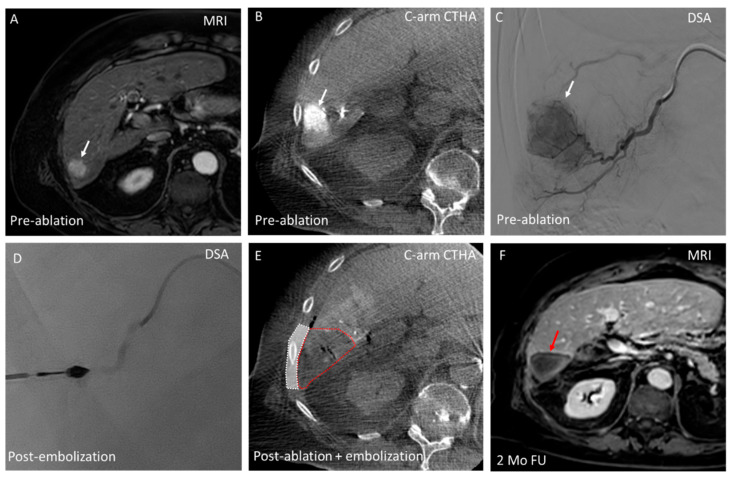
Combined bland embolization and ablation in a 55-year-old patient with a solitary HCC in liver segment 6. (A) The HCC (26 mm) (white arrow) is visualized on MRI (T1 + gadolinium) 2 months prior to the HepACAGA procedure; (B) Intraprocedural C-arm CT hepatic arteriography (C-arm CTHA) identifies the subcapsular lesion (white arrow) in close contact with the abdominal wall; (C) Digital subtraction angiography (DSA) demonstrates the target lesion (white arrow) and selective catheterization of the tumor-feeding artery using a 2.4 F Progreat microcatheter. PVA particles (150–250 µm) were injected to mitigate the heat sink effect before ablation; (D) Post-embolization DSA reveals contrast stasis in the tumor-feeding artery, confirming effective arterial occlusion prior to ablation; (E) Post-ablation C-arm CTHA confirms an adequate wedge-shaped ablation zone (outlined in red) after 5.5 min at 150 W. The adjacent abdominal wall (outlined in white) maintains its protective (heat sinking) arterial perfusion, preventing thermal injury; and (F) MRI (T1 + gadolinium) at 2-month follow-up (FU) demonstrates the wedge-shaped ablation zone (red arrow) with no evidence of local tumor recurrence.
